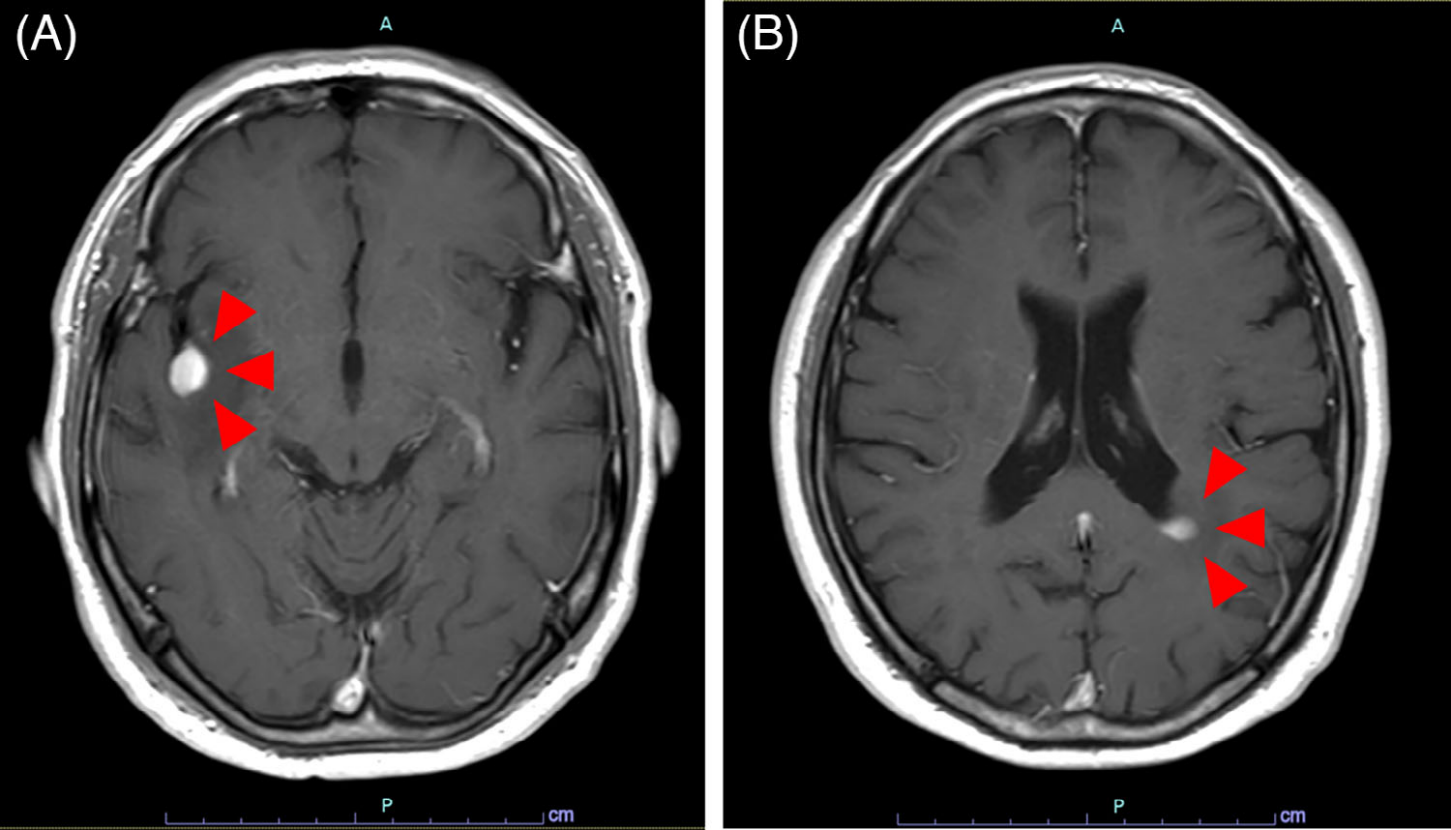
Gadolinium‐enhanced T1‐weighed magnetic resonance imaging shows mass lesions in the right insular lobe (A, red arrowheads) and the left periventricular white matter (B, red arrowheads).
